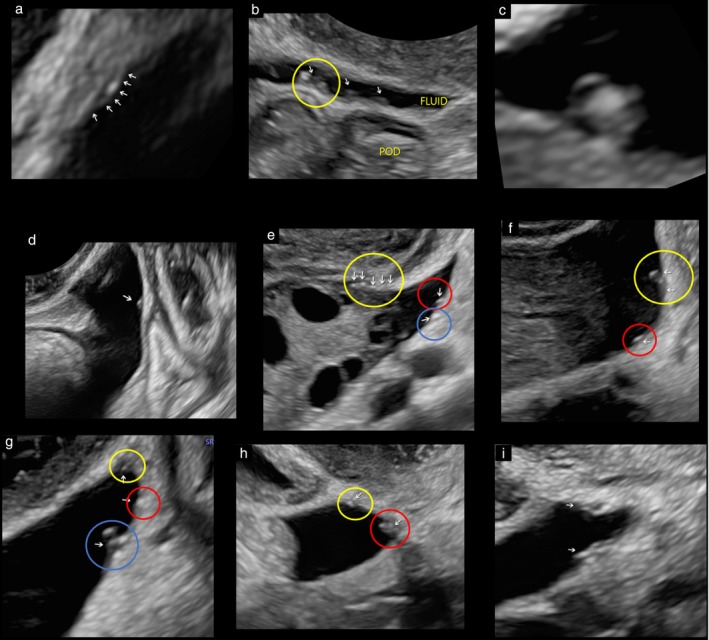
Examples of the ultrasound appearance of superficial endometriosis: (a) clustered linear cysts (arrows) with a hyperechogenic focus; (b) clustered tiny cyst (yellow circle and arrow) and two solitary cysts (arrows); (c) clustered tiny cysts with a honeycomb appearance; (d) solitary cyst (vesicle) (arrow); (e) clustered tiny cysts with a linear appearance (yellow circle and arrows), solitary tiny cyst (red circle and arrow) and solitary tiny cyst in a peritoneal small pocket (blue circle and arrow); (f) clustered tiny cysts with a honeycomb appearance (yellow circle and arrows) and solitary tiny cyst (red circle and arrow); (g) clustered tiny cysts with a hypoechogenic associated lesion (yellow circle and arrow), solitary cyst with a hyperechogenic focus in a small peritoneal pocket (red circle and arrow) and clustered cysts with a honeycomb appearance (blue circle and arrow); (h) solitary tiny cyst with hypoechogenic associated lesion and hyperechogenic focus (yellow circle and arrow) and clustered tiny cysts (red circle and arrow); (i) clustered cysts with a honeycomb appearance (arrows). POD, pouch of Douglas.
